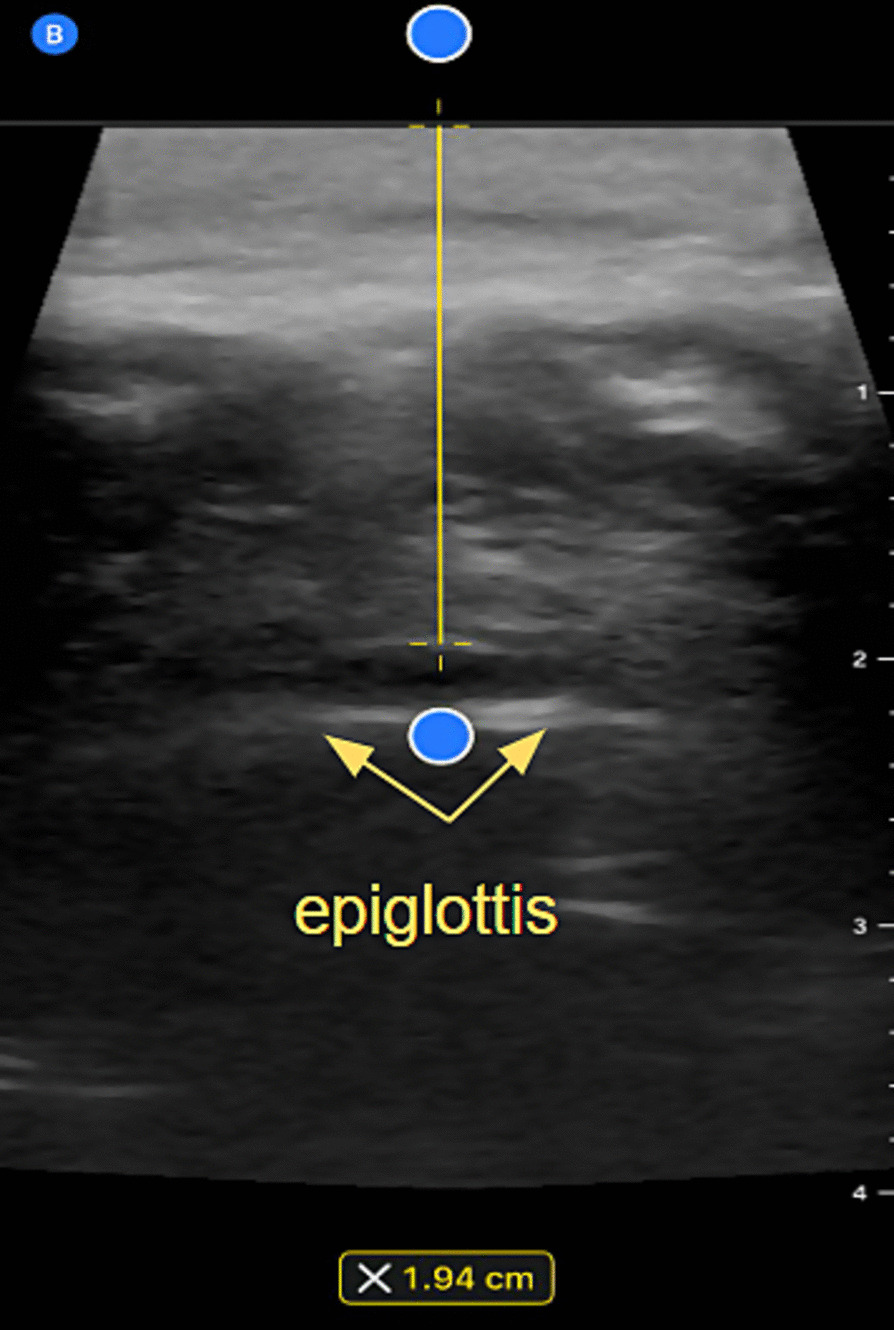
Airway assessment. A Upper Lip Bite Test. B Modified Mallampati Test. C Ultrasound assessment of skin-to-epiglottis distance
